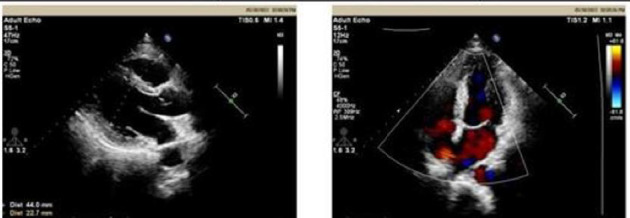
Cardiac ultrasound reveals no significant abnormalities detected in cardiac structure and blood flow for Case 1.
